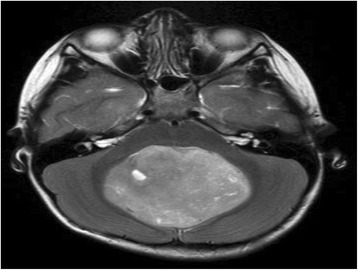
Contrast magnetic resonance imaging revealing an enhancing posterior fossa mass, involving the third ventricle: medulloblastoma
